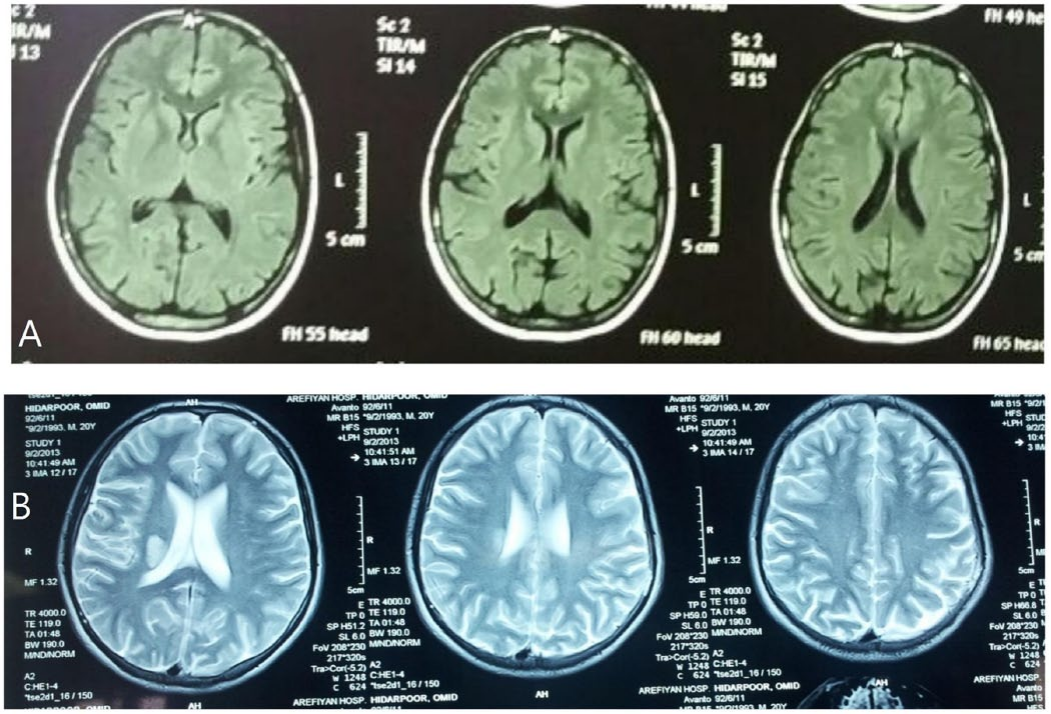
Brain magnetic resonance imaging of the patient showing (A) no abnormalities and (B) infarction of right periventricular region with extension to adjacent basal ganglia.
